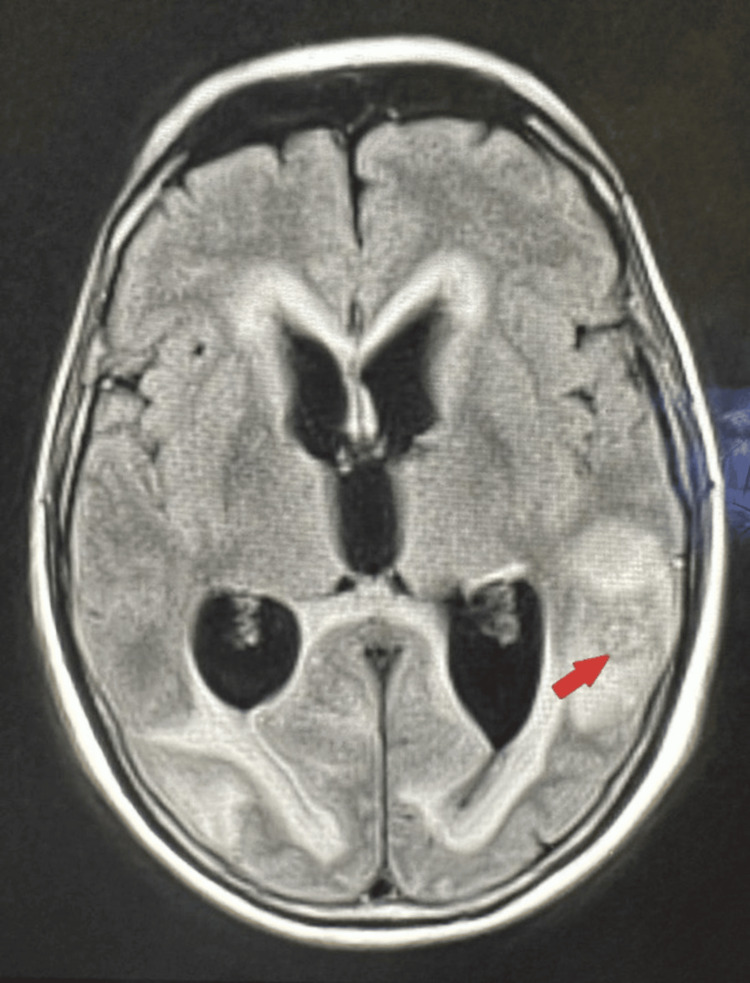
T2/FLAIR: periventricular hyperintensities, suggestive of trans-ependymal CSF seepage (red arrow) FLAIR: fluid-attenuated inversion recovery, CSF: cerebrospinal fluid
